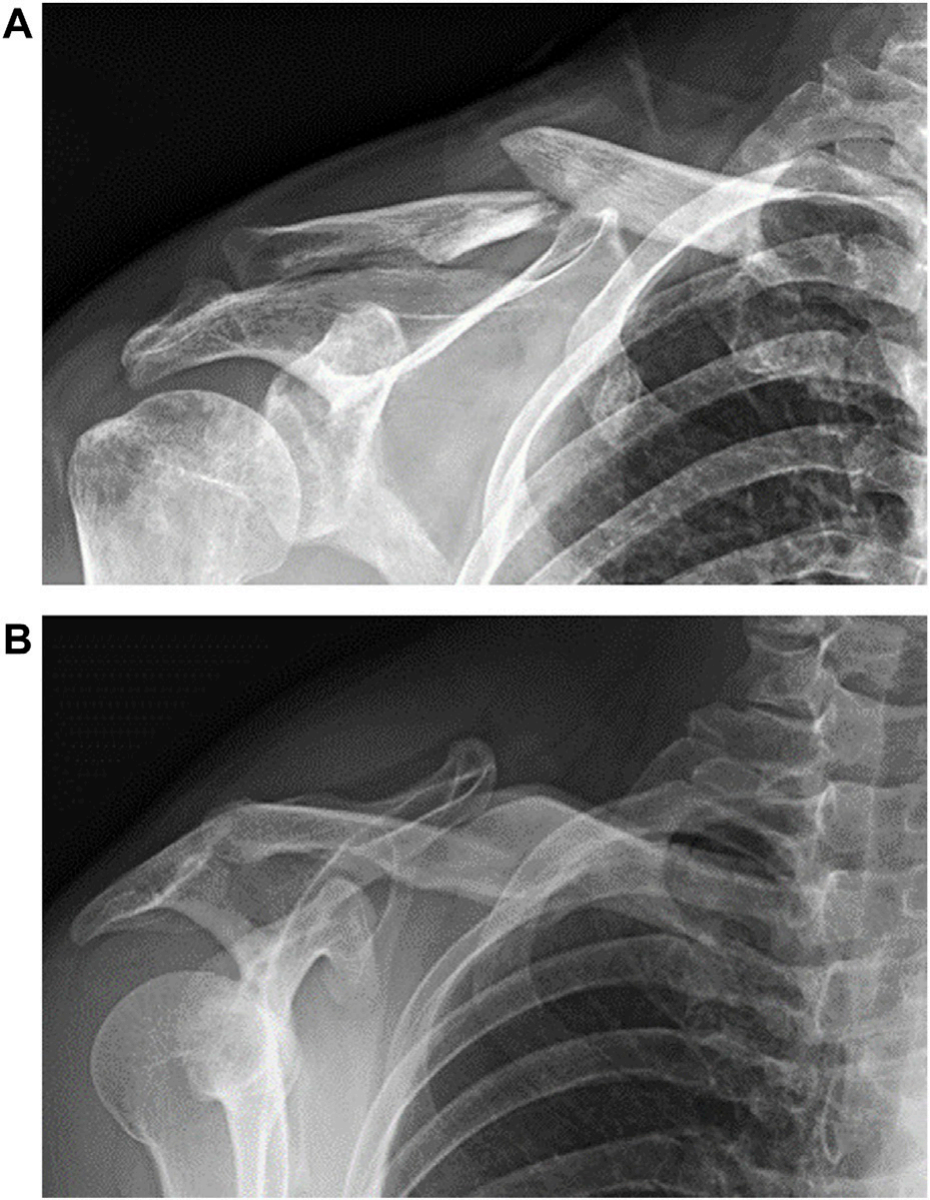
Radiographs of a typical mid-shaft clavicle fracture (A) before and (B) after treatment with a sling and a figure-of-eight bandage.
